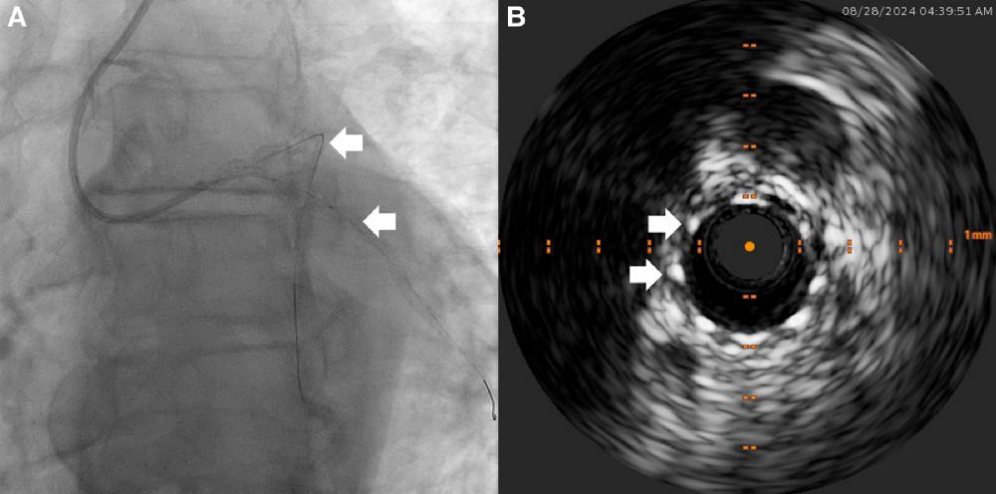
Intervention for Case 1, showing (*A*) the use of multiple guidewires (marked by arrows) to place the stents in the left circumflex and (*B*) intravascular ultrasound confirmation of stent placement in the left circumflex, with example stent struts identified by arrows (Supplementary material online, *Video S3*).
